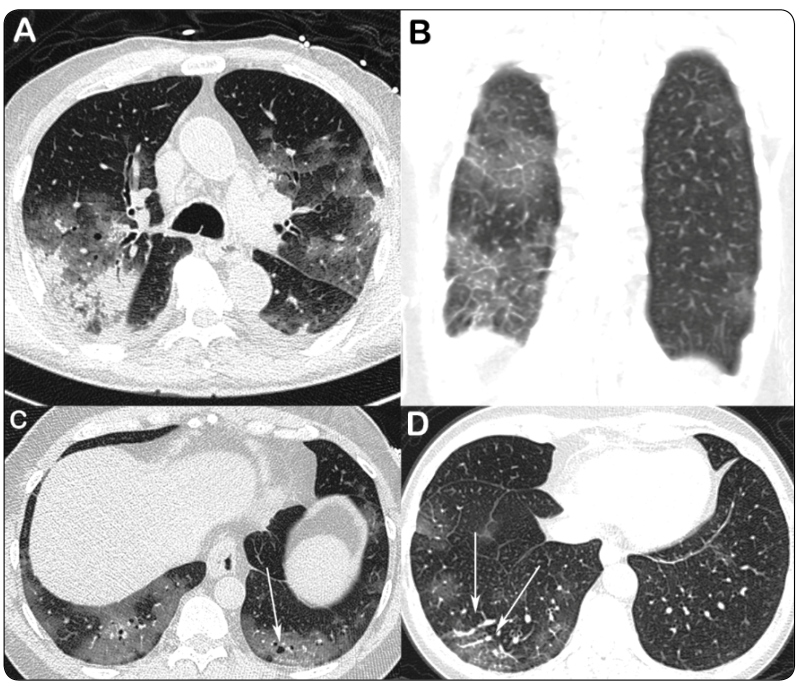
CT images obtained from four different patients with COVID-19 pneumonia. (A) Axial CT image of an 86-year-old man showing multiple bilateral patchy areas of ground-glass opacity. Also note peripheral areas of parenchymal consolidation in the right upper lobe. (B) Coronal reconstructed chest CT image of a 59-year-old man showing interlobular lines superimposed on a background of ground-glass opacity (crazy-paving pattern) in the right lung. (C) Axial CT image of a 56 year-old-man depicting tiny cystic air spaces (arrow). (D) Axial CT image of a 64-year-old woman showing vascular dilatation associated with ground-glass opacities in the right lower lobe (arrows).
